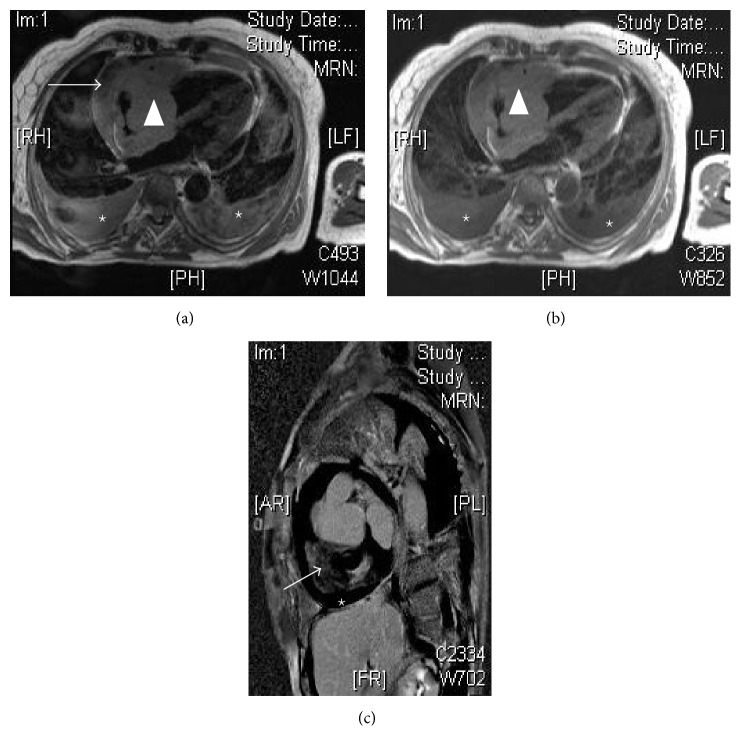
Cardiac magnetic resonance imaging (MRI). (a) Axial T2 cardiac section, showing an isointense homogeneous mass with respect to the RA myocardium (arrowhead), with pericardial effusion (arrow) and bilateral pleural effusion (star). (b) Axial T1 section, similar to the previous image, showing an isointense homogeneous mass with respect to the myocardium that occupies almost all of the RA, without fat infiltration (arrowhead) and bilateral pleural effusion (star). (c) shows the short-axis RV with delayed gadolinium enhancement and a pattern of diffuse and ill-defined enhancement (arrow). Pericardial effusion (star).
